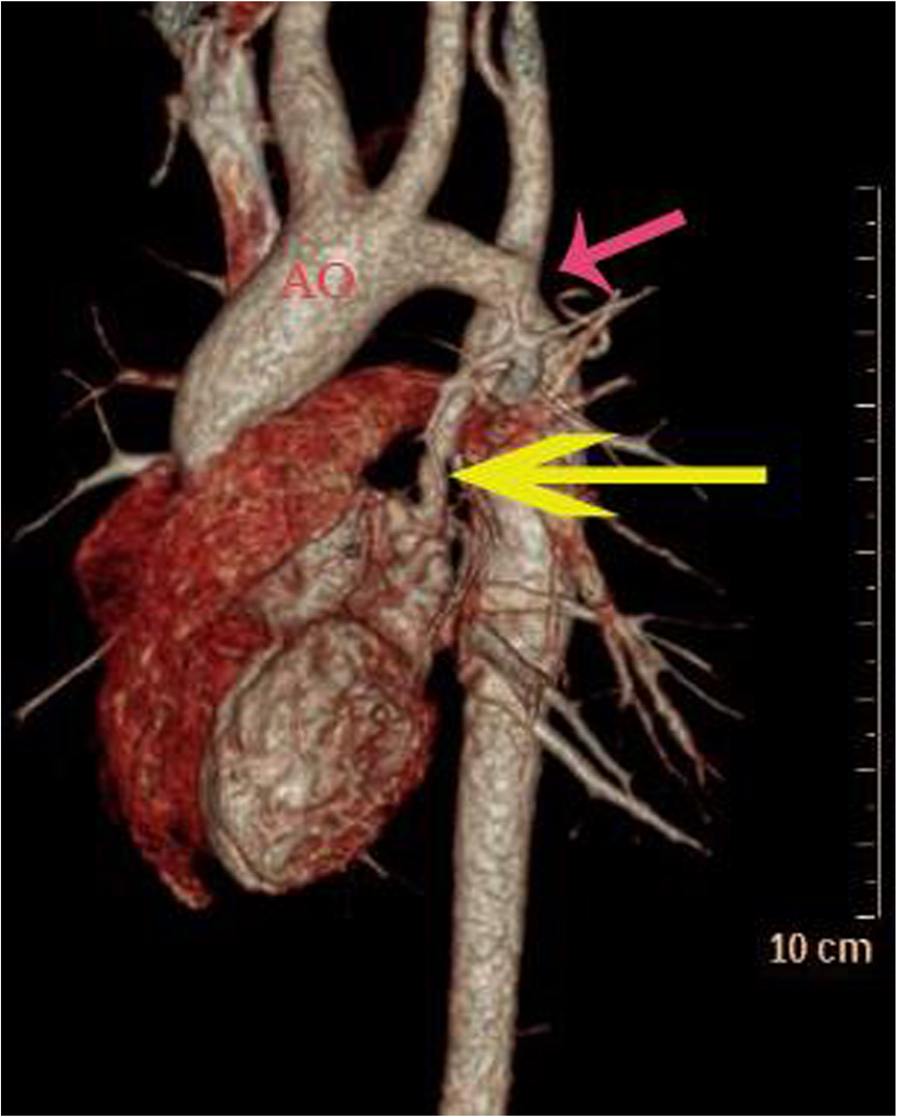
Postoperative computed tomography demonstrating the left superior pulmonary vein draining into the left atrial appendage (AO = aorta; *red arrow* = correction of the coarctation; *yellow arrow* = left superior pulmonary vein draining into the left atrial appendage)
